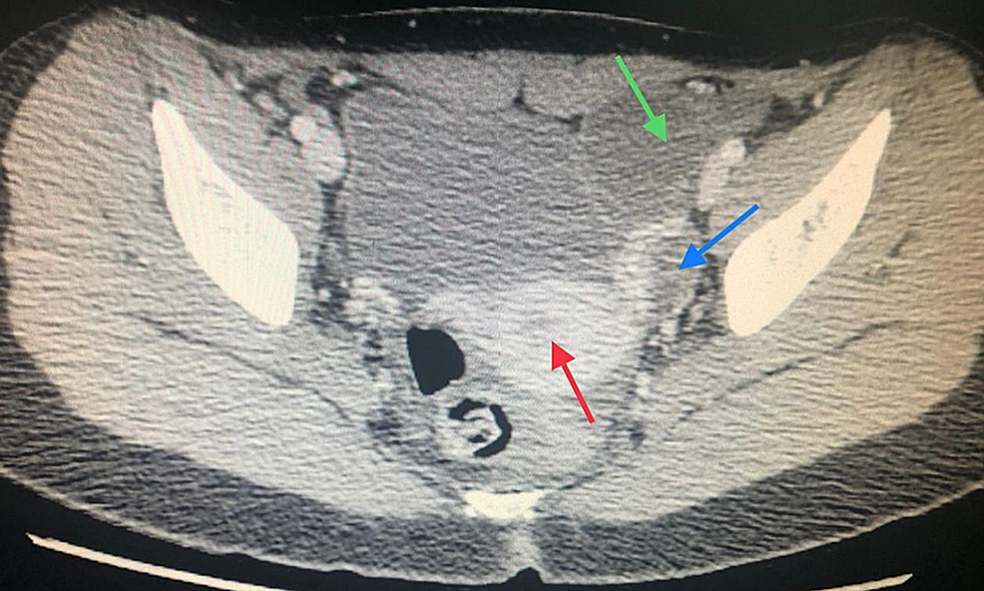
CT scan pelvis with contrast shows left paraovarian cyst (green arrow), uterus (red arrow) and left ovary can be visualized separately (blue arrow).
